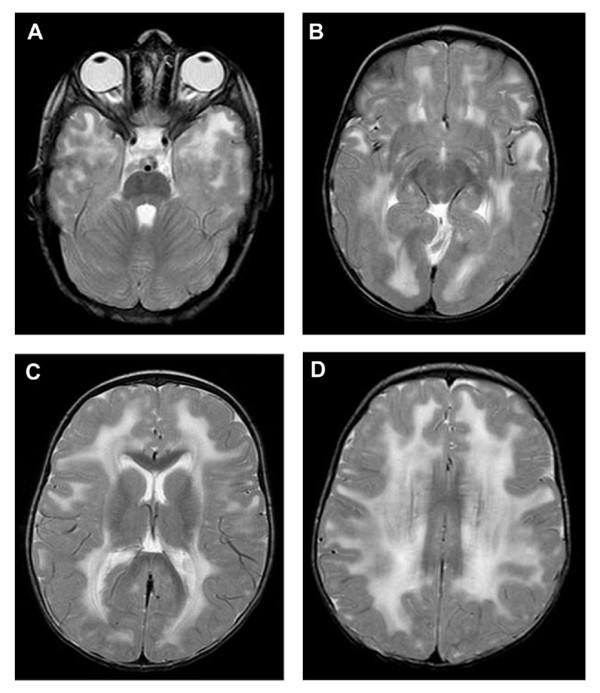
T2-weighted MRI images of patient 7, taken at the age of 19 months. Showing abnormal periventricular and sub-cortical white matter signal.
